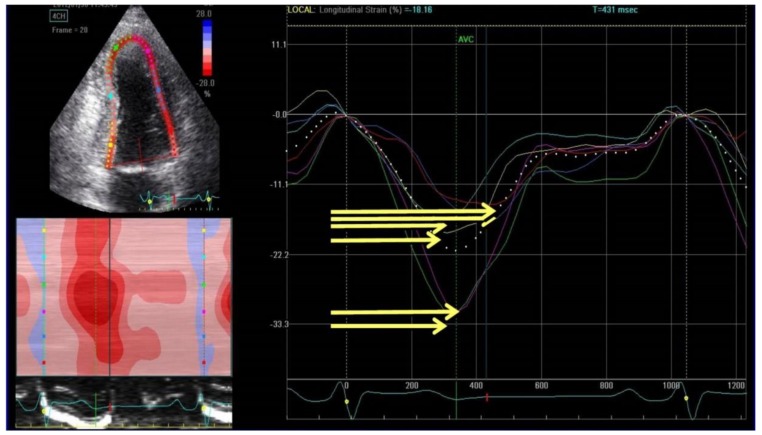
Evaluation of mechanical dispersion by speckle tracking echocardiography. Mechanical dispersion is calculated as the standard deviation of time to peak regional negative strain (yellow lines).
